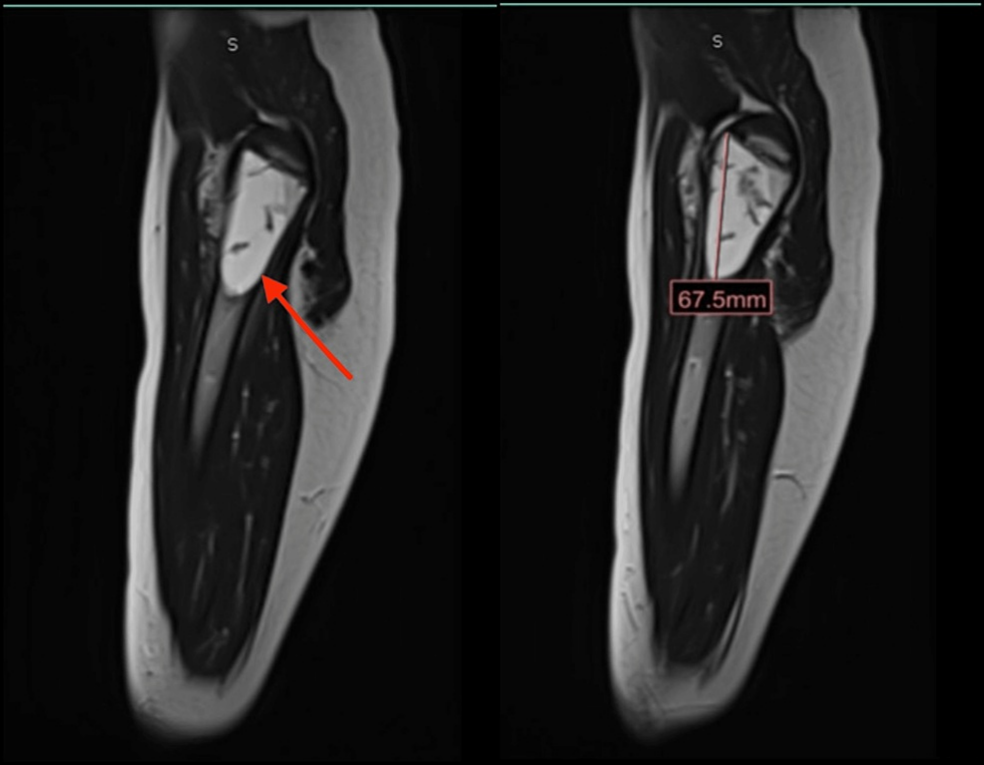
MRI showing a multiloculated expansile lesion of the left femoral neck with areas of layering hemorrhage and pathologic fracture
